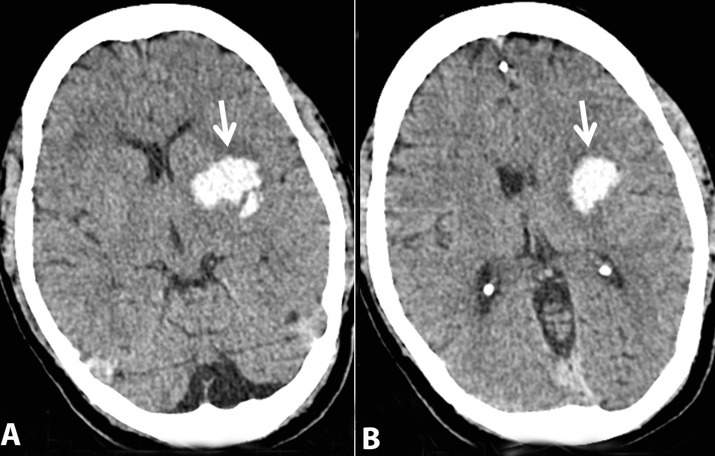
Axial non-contrast CT scan images of the brain showing a hematoma in the left gangliocapsular region (arrows).
